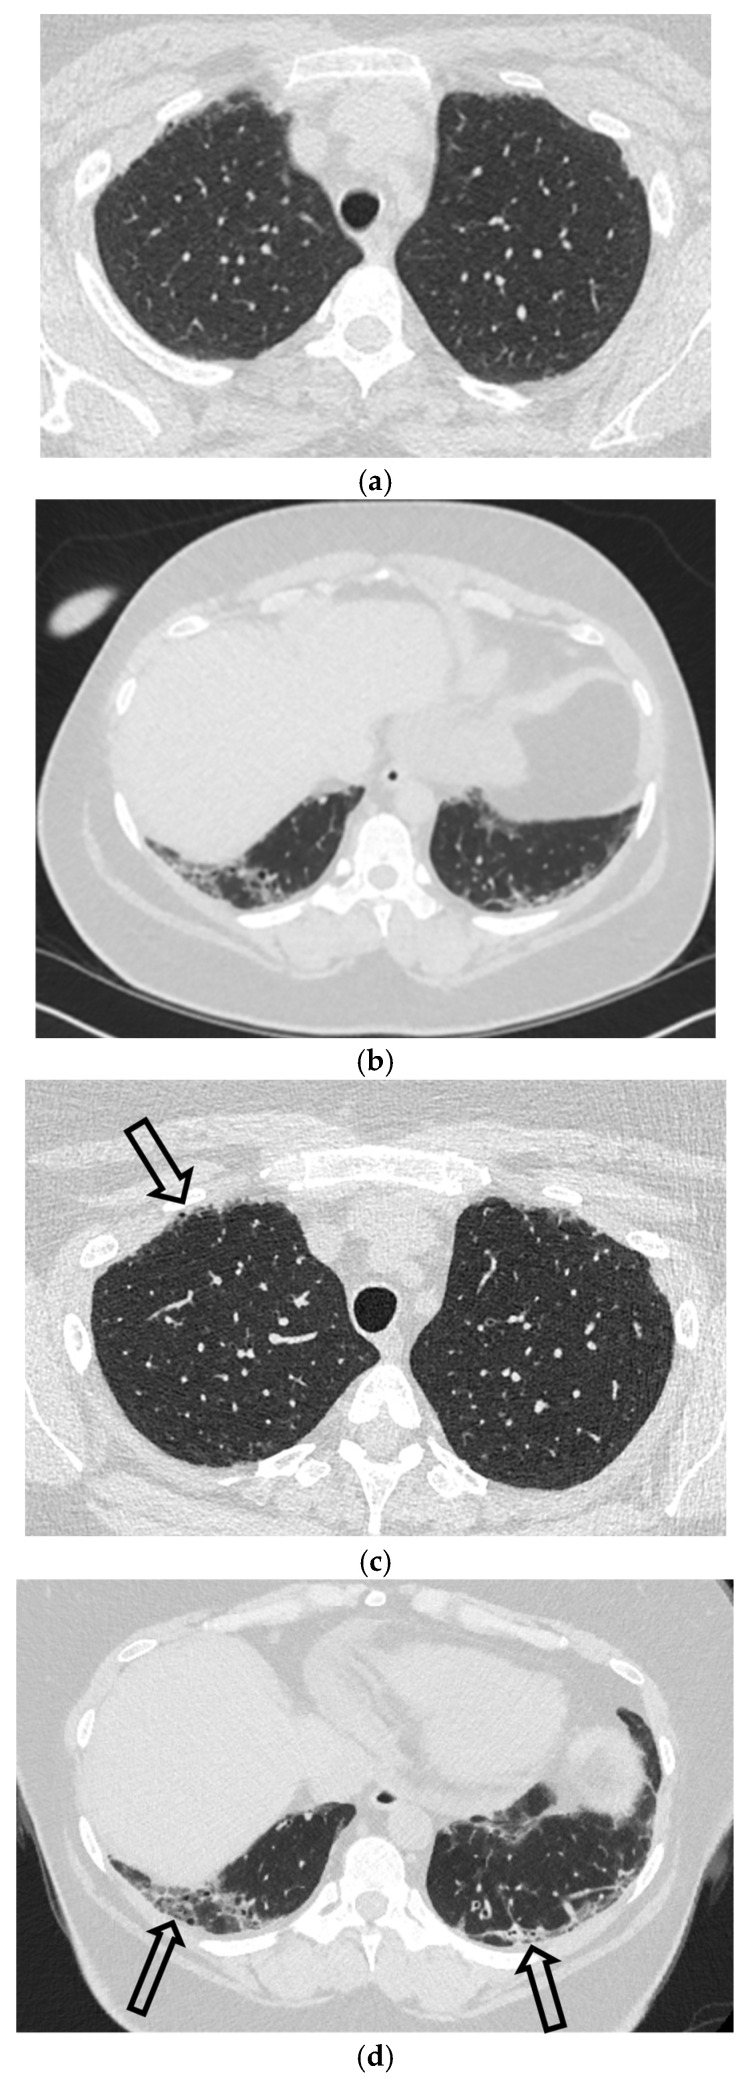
38-year-old female with systemic lupus erythematosus. Axial EID-CT images in lung window (a,b) and axial PCD-CT images in lung window obtained about 1 year later (c,d) demonstrate peripheral basilar-predominant ILD (open black arrows) consistent with NSIP. Note improved visualization of fine peripheral linear interstitial opacities, associated ground-glass opacities, and mild traction bronchiolectasis on PCD-CT images compared to EID-CT images.
